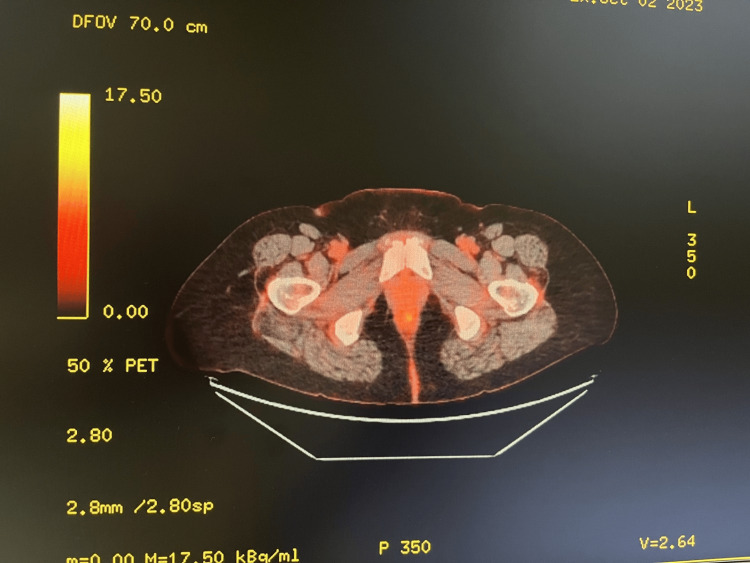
Positron emission tomography-computed tomography (PET-CT) Transverse plane. Cervical tumor with heterogeneous, mildly increased metabolic activity
